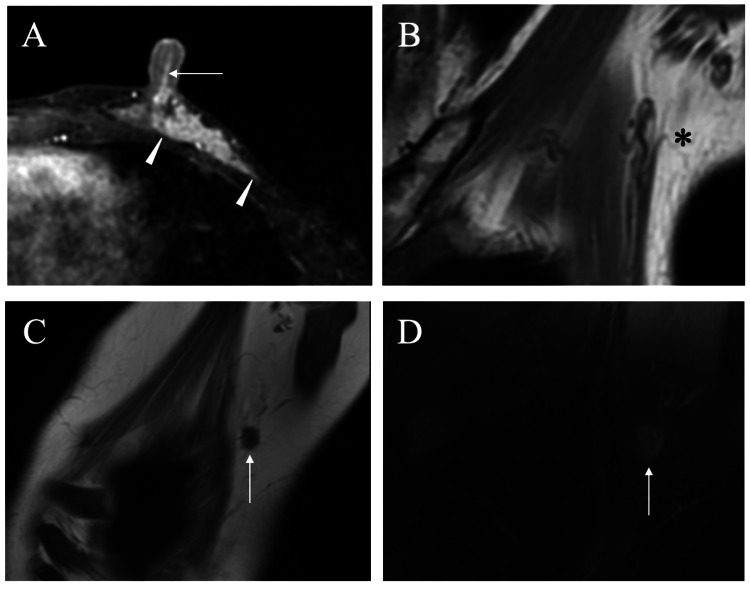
Magnetic resonance imaging (MRI) findings A. Subtraction MRI at the primary operation showed extremely wide enhancement, i.e., more than 40 mm (arrowheads), in the left mammary gland including intra-nipple ducts (arrow) by presumed ductal spread. B. MRI at the primary operation did not show any mammary glands in the axilla (asterisk). C. MRI of the axillary mass showed low signals on T1-weighted images. D. T2-weighted MRI images of the axillary mass only showed faint high signals.
